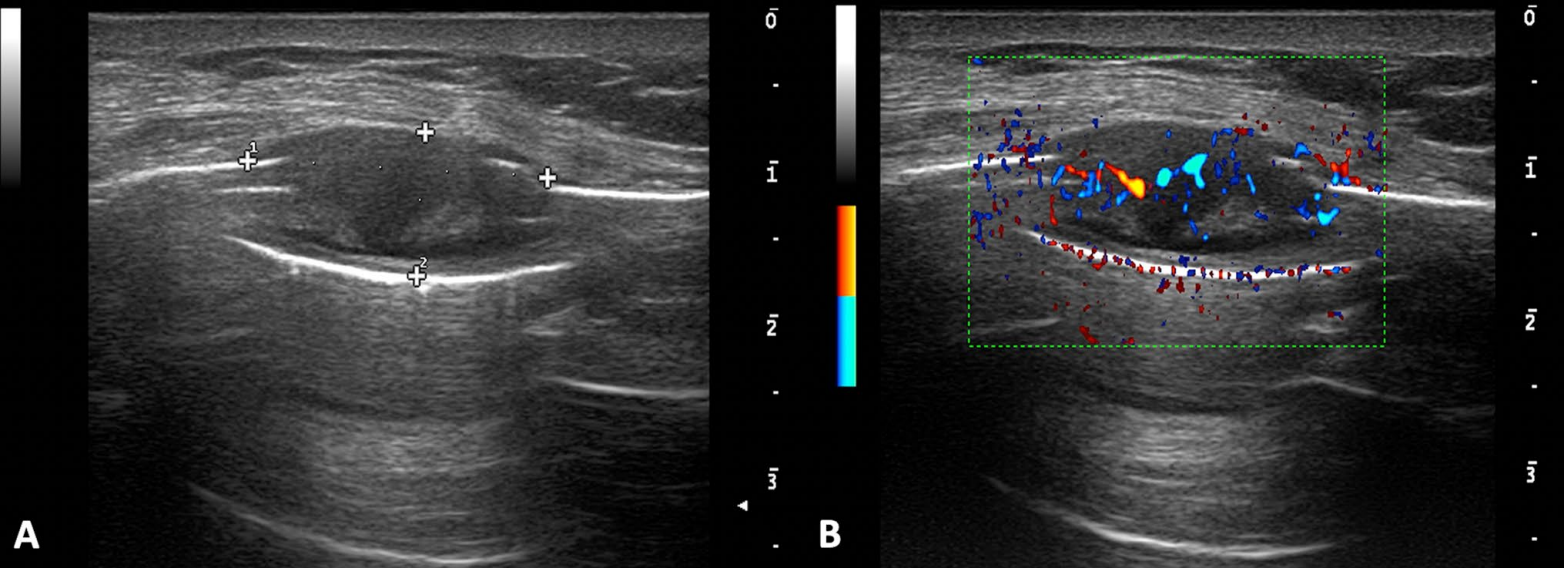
Female patient of 55 years old. B-mode (A) and color Doppler (B) scans. US reveals a heterogeneous, hypoechoic rib metastasis in patient with mastectomy, which disrupts echogenic cortical line. Color Doppler shows rich intralesional vascularization (B)
